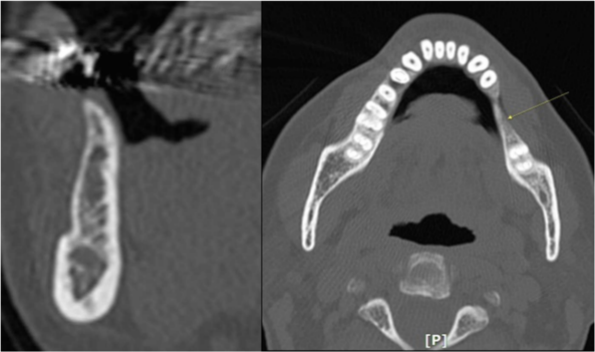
CBCT, preoperative view. The alveolar bone of #35, 36 (tooth loss state) was deficient severely. It needed bone graft to increase the width of alveolar bone where implant could be placed
